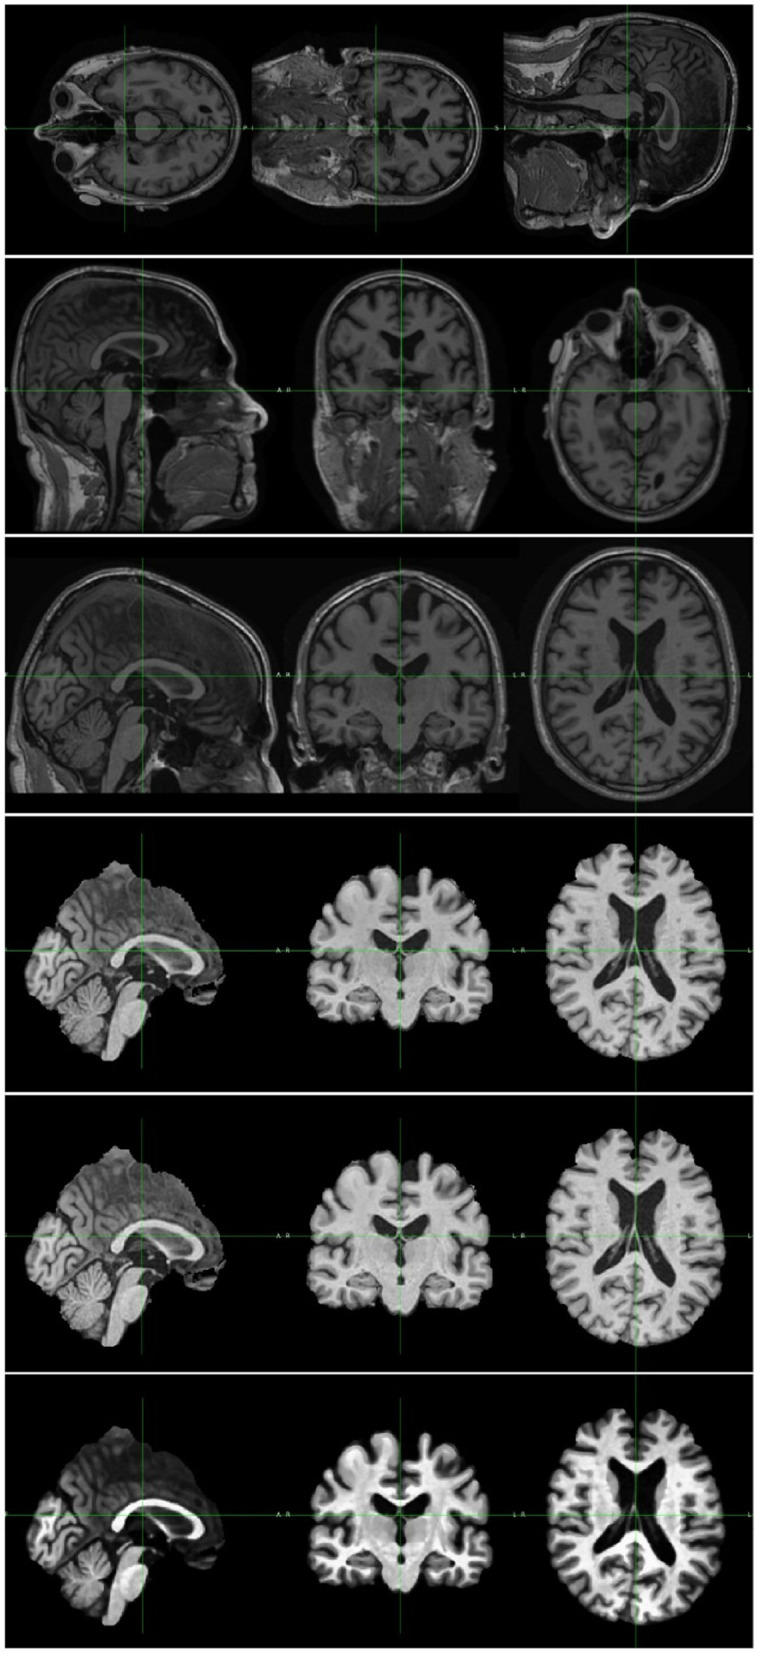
Image pre-processing sample. The top image is the original MRI scan. The rest are samples of image after each preprocessing step, including, orientation, registration, skull stripping, bias field correction, and enhancement.
